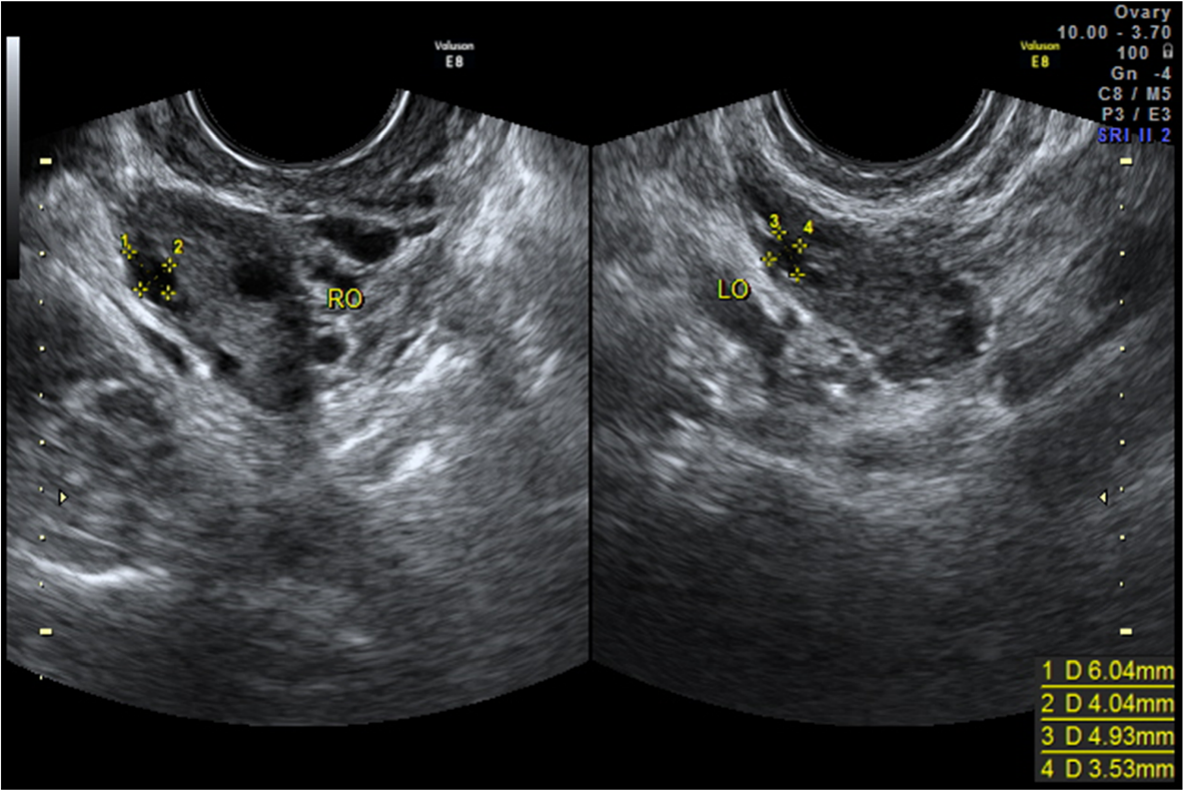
Transvaginal ultrasound scans of two ovaries with normal number of antral follicles, in line with normal AMH levels but in contrast with high serum FSH and LH
